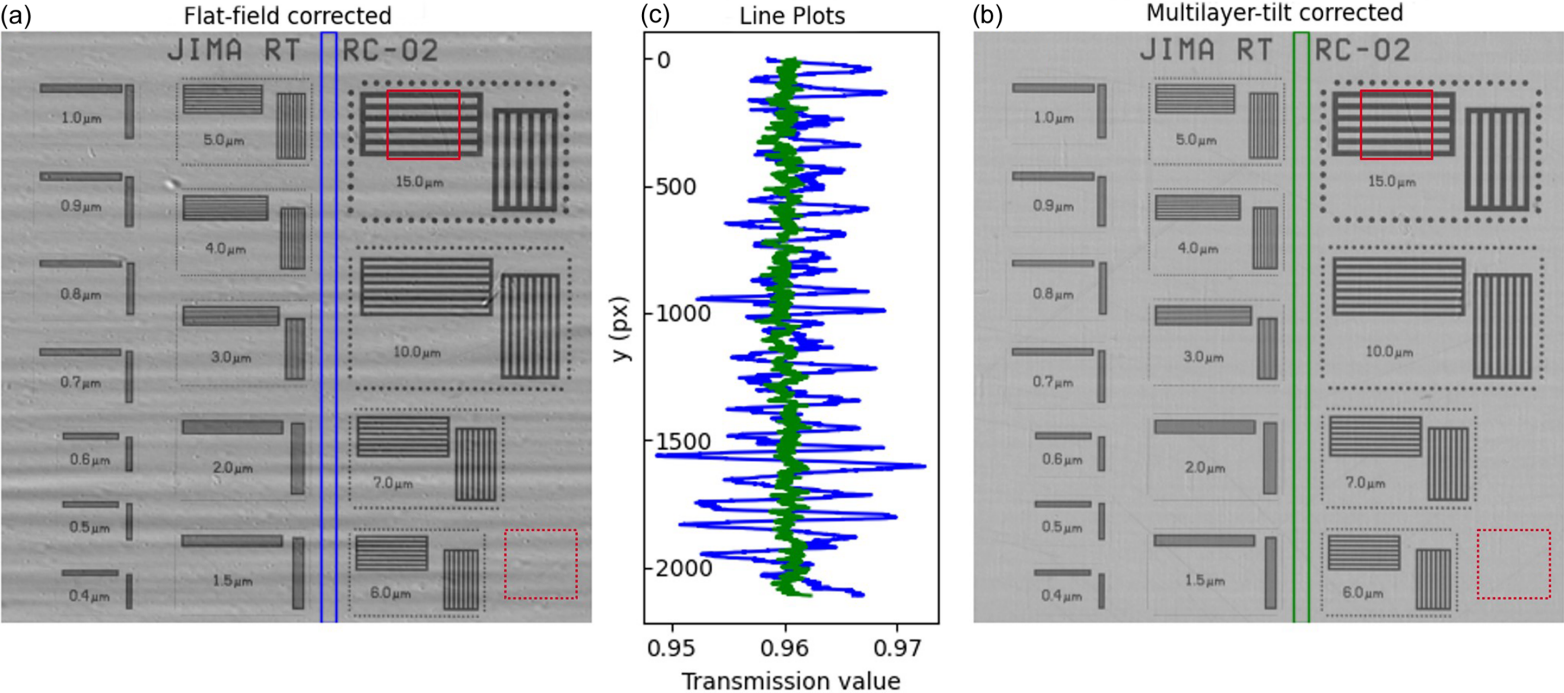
(*a*) Static flat-field correction *I*_static−FF_ of 10× radiography of the JIMA RT RC-02 target. (*b*) Dynamic flat-field correction *I*_DMM − FF_ of the same FOV and (*c*) transmission values plotted through both images in the same empty region (averaged over a 50 pixel line width). Both radiographs were taken at 25 keV and at a distance of 15 mm from the detector. The red rectangles in (*a*) and (*b*) show the ROIs used for CNR/SNR calculations, centered on tungsten lines (solid rectangle) and on the background (dashed lines).
